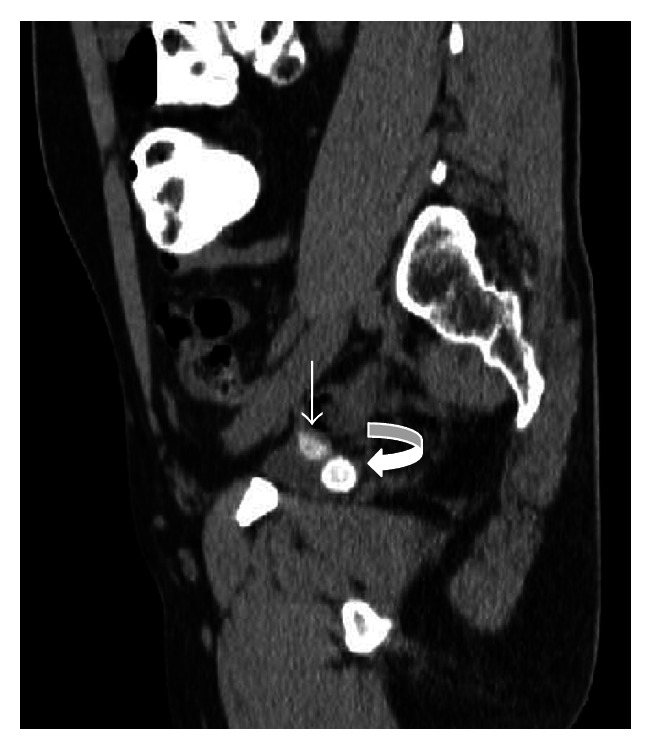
In CT images of the patient, bladder is not full enough but 2 cm hyperdense calculi are seen in posterior inferior part of bladder (curved arrow) and hyperdense fecalith image is seen in right superior posterolateral part of bladder (arrow).
